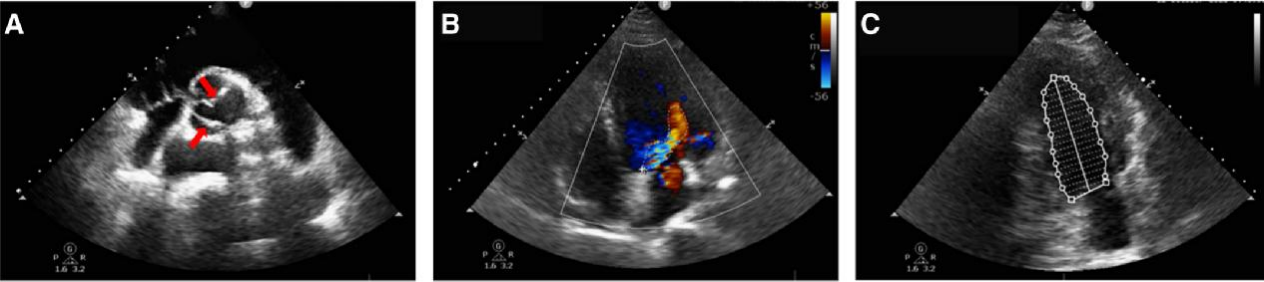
Transthoracic echocardiography showed bicuspid malformation (arrows) (*A*), regurgitation (regurgitation area of 7.0 cm^2^; dotted box) of the aortic valve (*B*), and the systolic dysfunction (biplane Simpson’s method: ejection fraction 44.0%) of the left ventricle (*C*).
